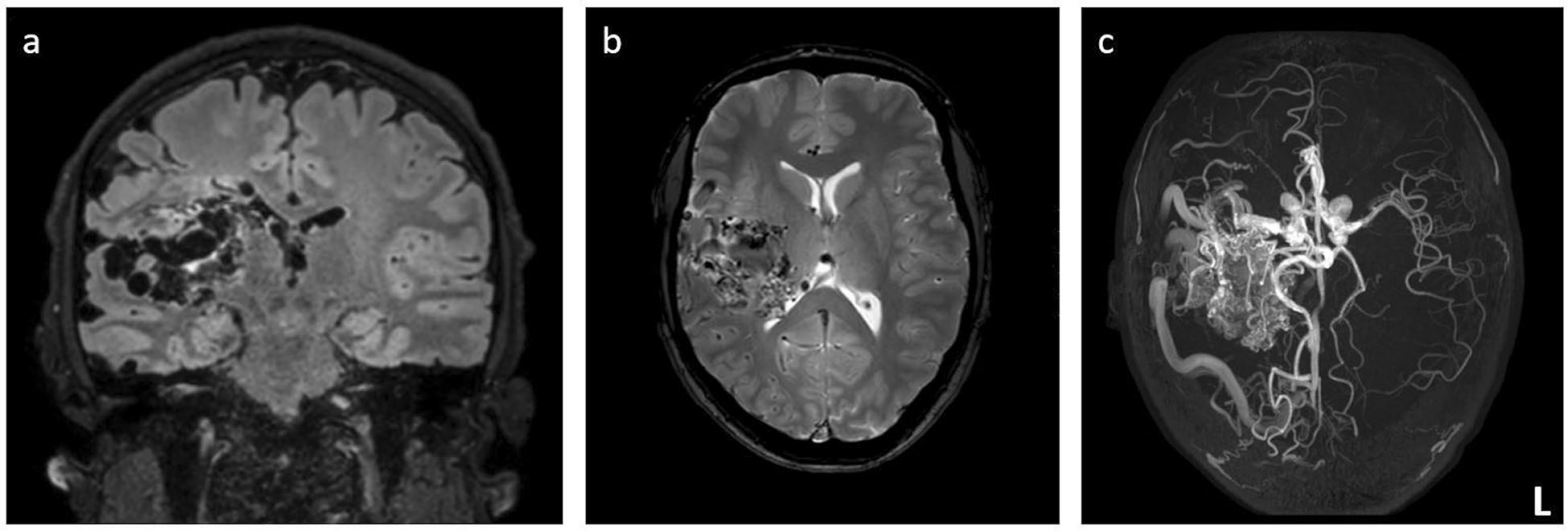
Structural magnetic resonance imaging (MRI) and conventional magnetic resonance angiography (MRA). Coronal fluid attenuated inversion recovery (FLAIR, a) shows a large space-occupying lesion suggestive of an arterio-venous malformation (AVM) with compression of the right lateral ventricle. According to axial T2*-weighted imaging (b), no evidence of bleeding was revealed. Time-of-flight (TOF)-MRA in a paraxial view (c) shows an increased arterial flow signal in the right hemisphere with evidence of dilated draining venous structures containing arterialized flow
